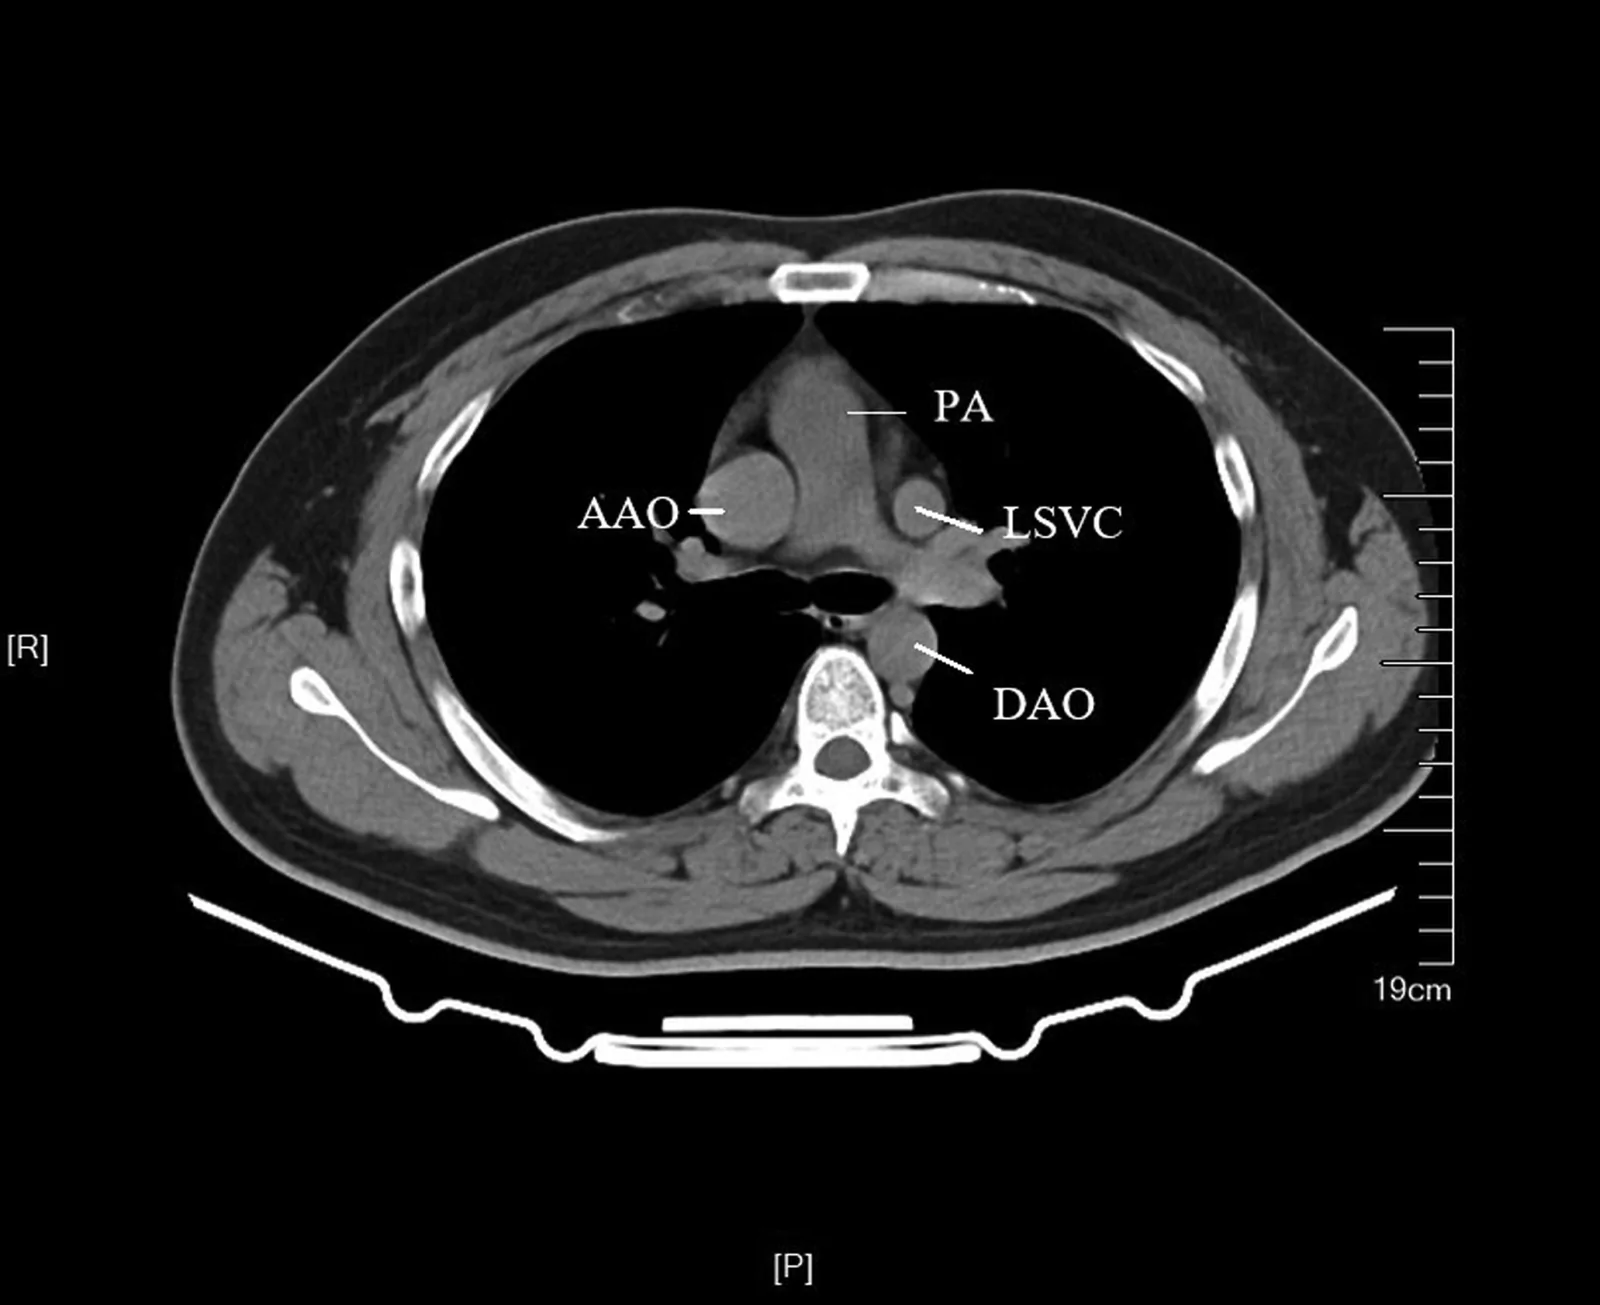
Axial section of CT thorax at (mediastinal window) showing left-sided superior vena cava. AAO = ascending aorta, DAO = descending aorta, LSVC = left superior vena cava, PA = pulmonary artery.
